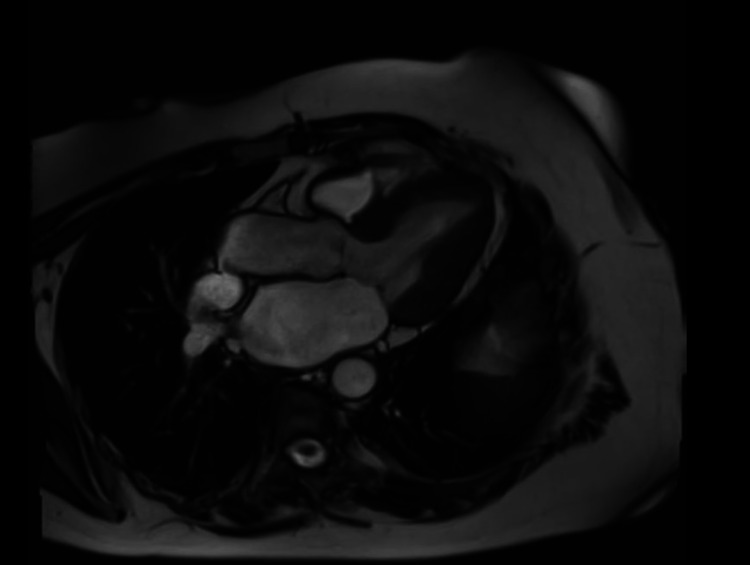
Cardiac MRI scan performed 3 months after discharge from hospital shows normal contractility of the left ventricle, along with resolution of apical ballooning.
